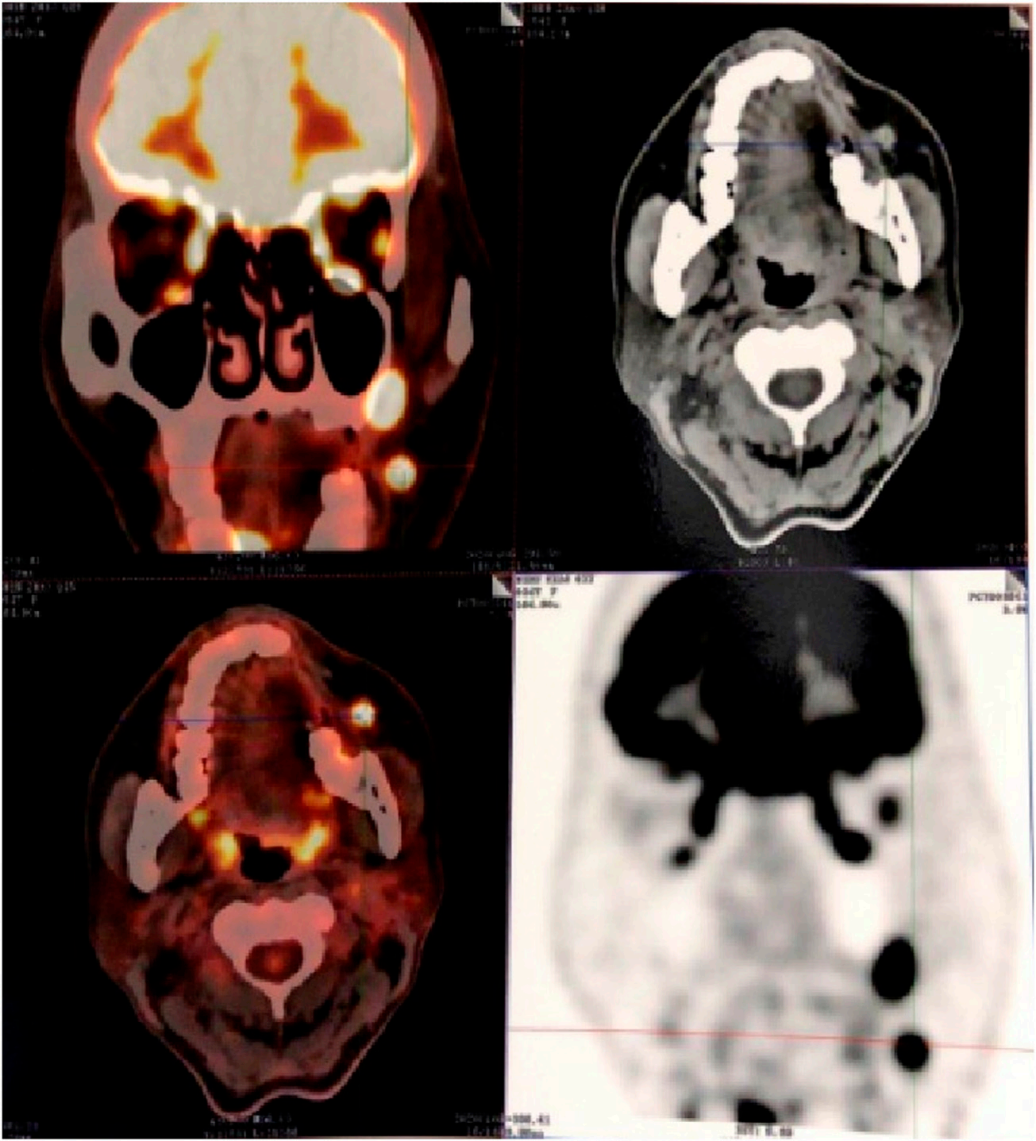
PET-CT axial view shows metabolic hyperactivity of the lymph node mass on the left side.
